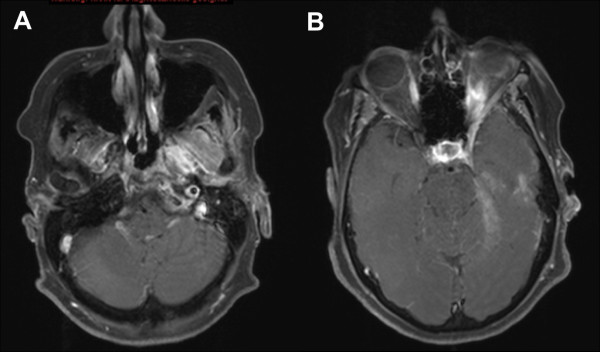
Follow-up contrast enhanced MRI 14 months post radioimmunotherapy: therapy-related changes: pterygoid muscles (a) and cavernous sinus/left orbit (b).
